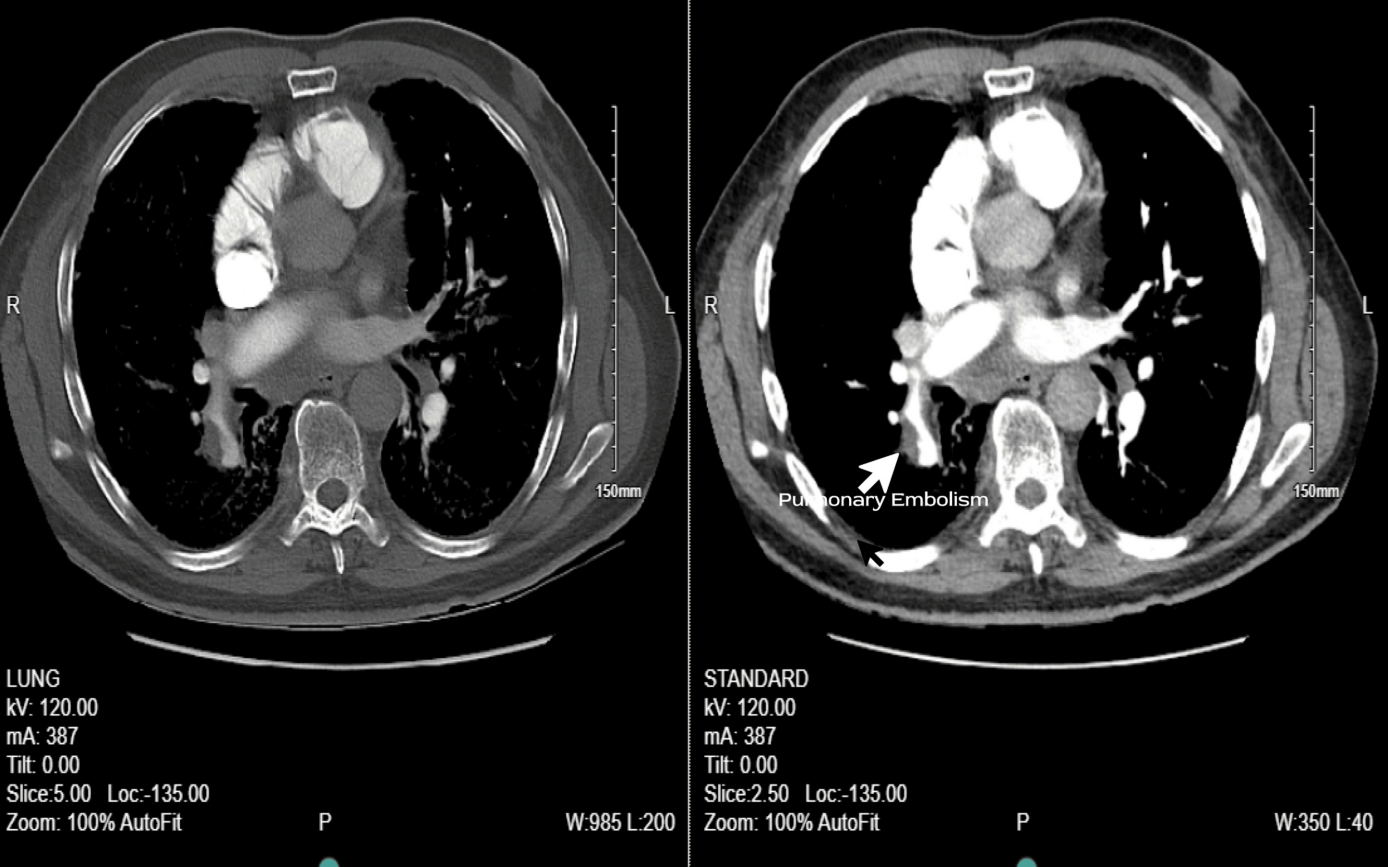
CT angiogram of pulmonary embolism with contrast Acute-on-chronic pulmonary embolism involving the right lower lobe pulmonary arterial segmental branches, with evidence of right heart strain and pulmonary arterial hypertension.
